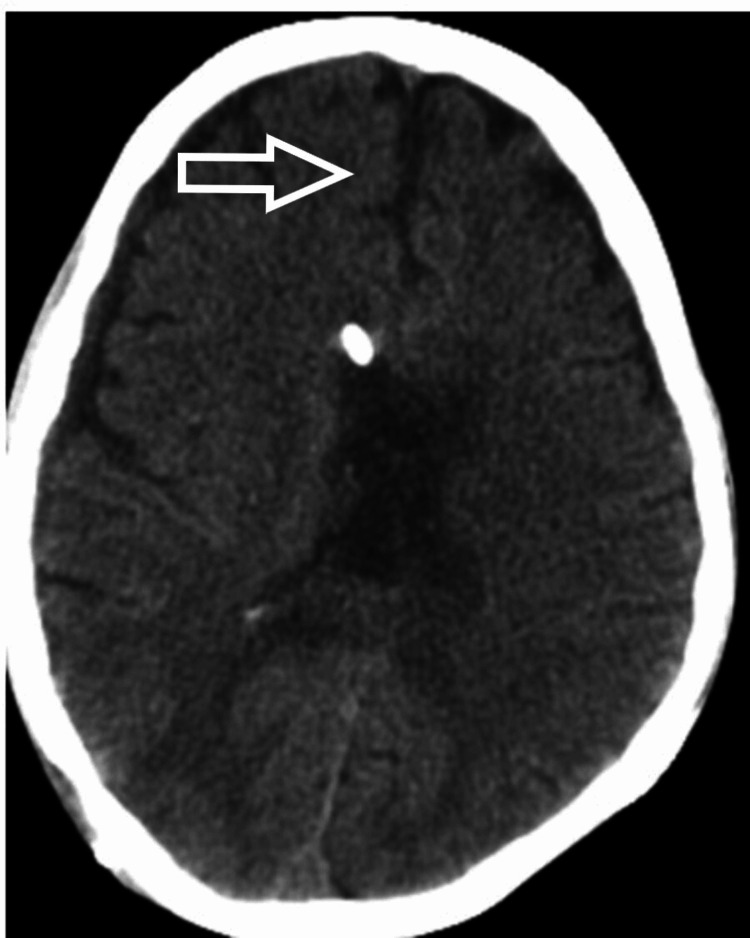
NCCT head showing hydrocephalus and midline shift - view 2 NCCT: noncontrast computed tomography
